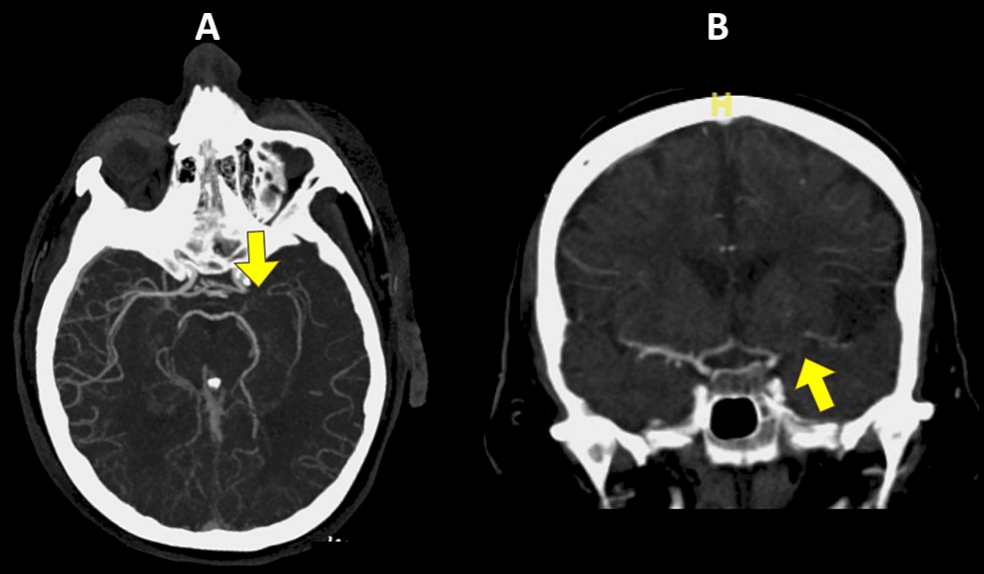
Successful identification by Viz LVO A 67-year-old female patient presented with left M1 MCA occlusion after a fall. The admission NIHSS score was 15. She was successfully revascularized with thrombectomy. The 24-hour post-intervention NIHSS score was 3. A) Viz LVO identification of M1 occlusion (arrow) on the axial view of CT angiography helical reconstruction. B) Coronal view of non-contrast CT angiography with M1 occlusion (arrow). LVO, large vessel occlusion; MCA, middle cerebral artery; M1, M1 segment of the middle cerebral artery; NIHSS, National Institute of Health Stroke Scale; CT, computed tomography.
